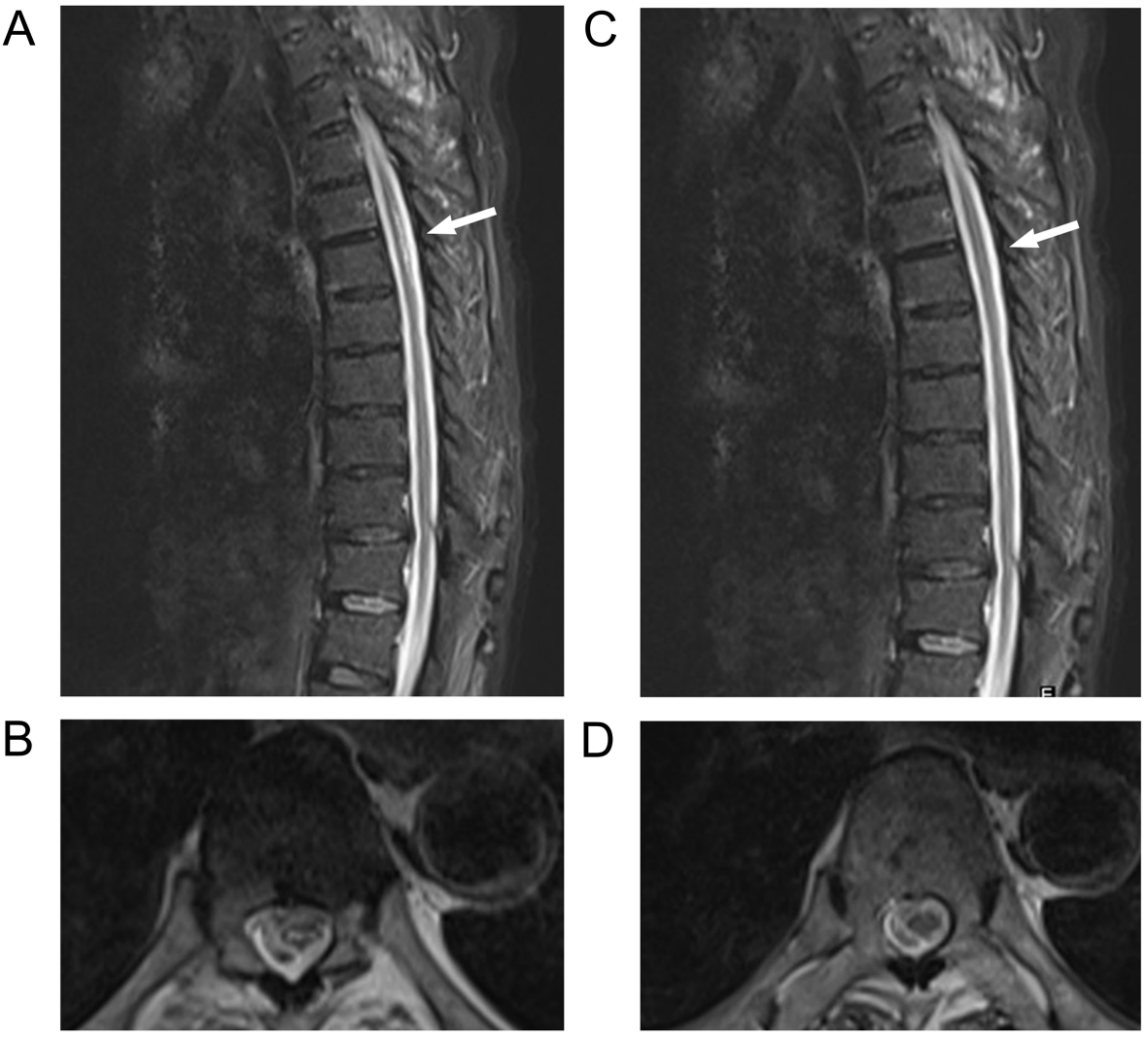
T_2_-weighted magnetic resonance images of thoracic vertebra in Case 1 at the time of the first (A and B) and last admissions (C and D). A, Sagittal view shows the thoracic spinal cord with a high-signal focus (arrow). B, Axial view shows the thoracic spinal cord and the “small print” sign (at the T5-T6 level; arrow). C, Sagittal and D, axial views show that the thoracic spinal cord lesions had partially recovered (at the T5-T6 level; arrows).
